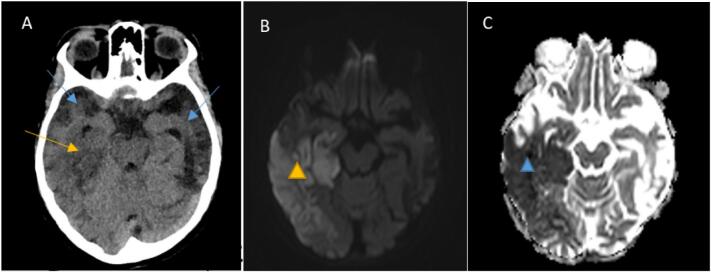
Selected axial brain images for our patient, brain CT scan Brain MRI (A) axial brain CT scan cut is showing bitemporal lobe atrophy (blue arrows) as well as right posterior temporal lobe hypodensity suggestive of early ischemic changes. Corresponding MRI images, (B) diffusion sequence demonstrates right temporoparietal cortical and subcortical area of high signal (yellow triangle) with corresponding image low signal seen at (C) ADC sequence (Apparent diffusion coefficient) (blue triangle) in keeping with acute infarction.
